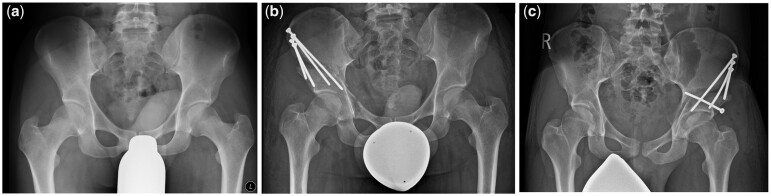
(a) Preoperative radiograph. (b) Post-operative radiograph after PAO without transverse screw fixation. (c) Post-operative radiograph this time with use of a transverse screw.
